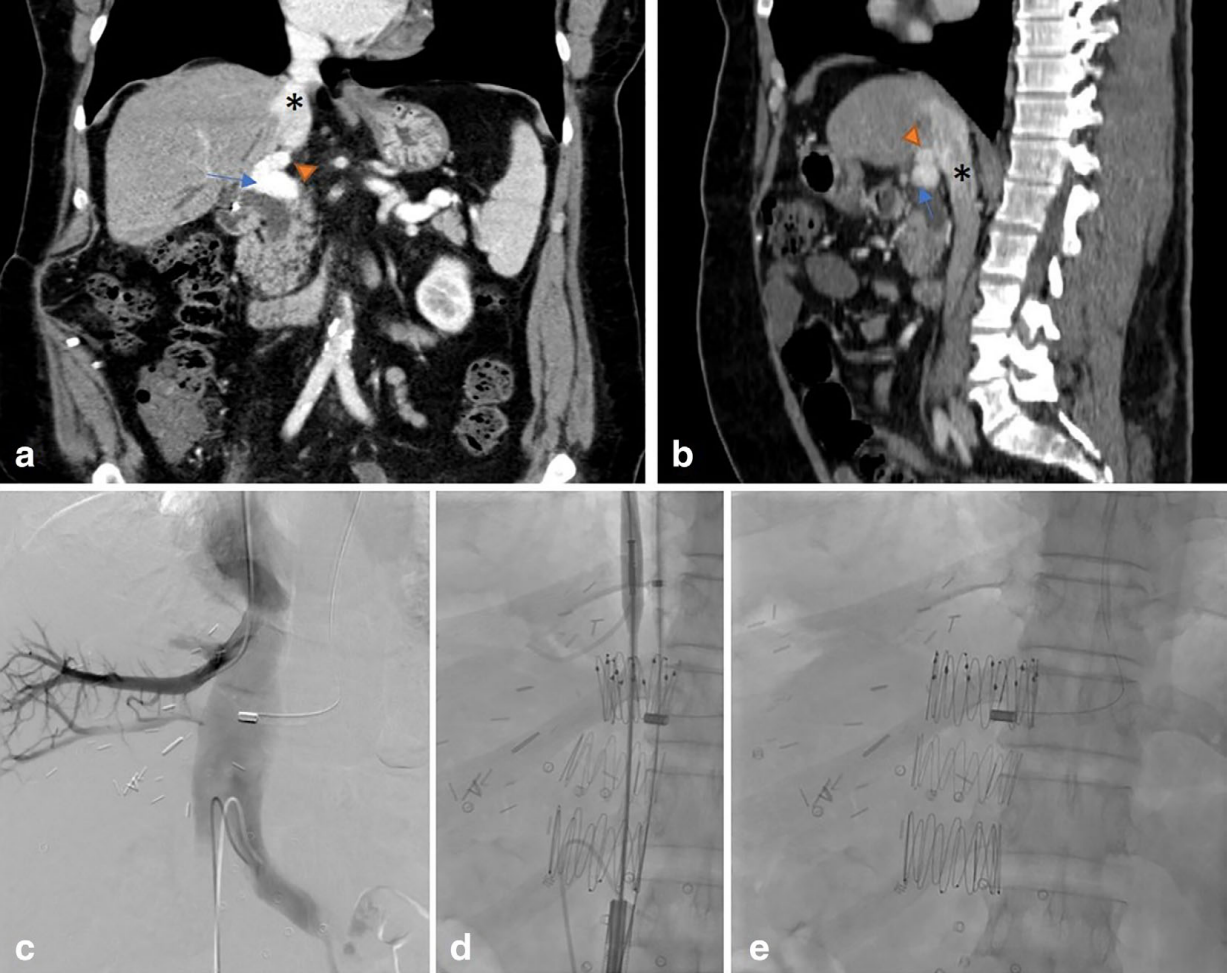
(a, b) Coronal and sagittal Maximum Intensity Projection CECT images showing a surgically created hemi‐portocaval shunt (orange triangle) between the IVC (*) and the right portal vein (blue arrow). Reformatted CT images were used to estimate the relation of the hepatic venous outflow and the Portocaval shunt outflow into the IVC to guide the IVC stent deployment. (c) Combined hepatic and IVC venogram was done to assess the landmarks for IVC stent placement and correlate it with pre‐defined CT measurements. (d, e) Metallic IVC stent graft deployed over a guidewire to obliterate the portocaval shunt. IVC, inferior vena cava.
